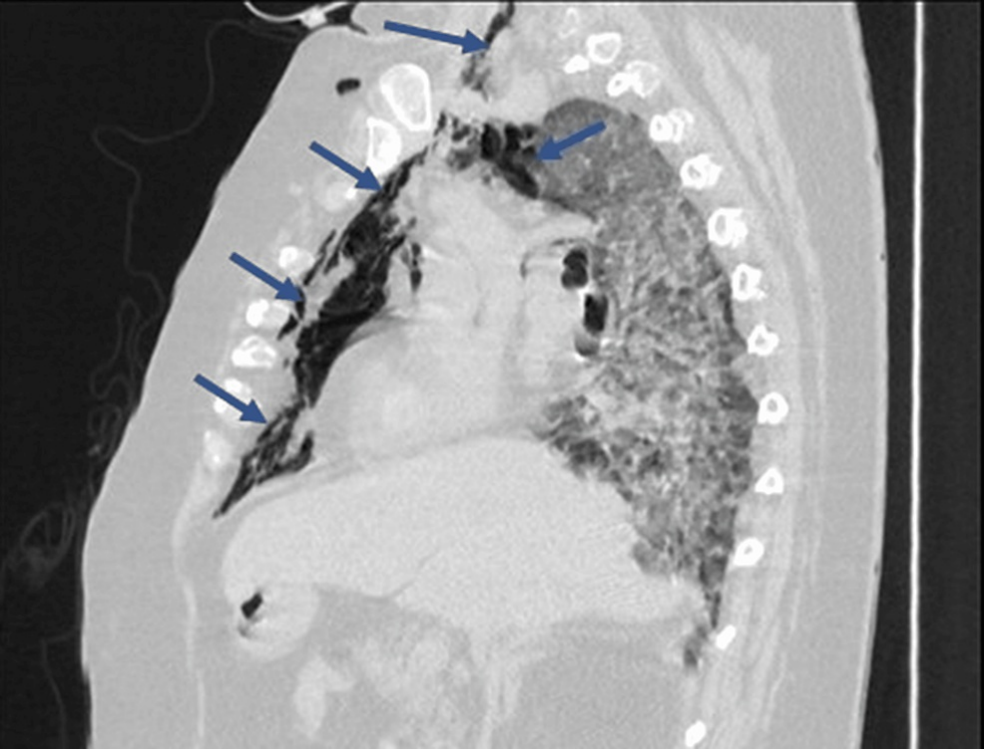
Computed tomography of the chest (sagittal section) Pneumomediastinum and subcutaneous emphysema are observed in this image.
